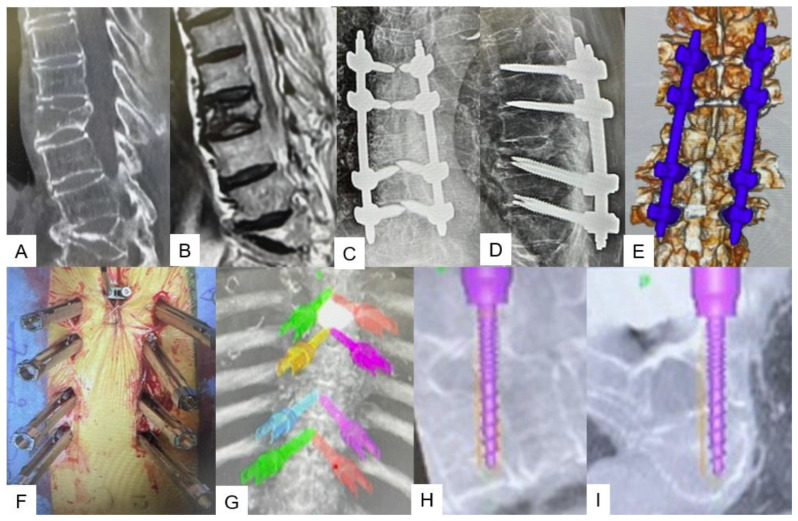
T9 extension fracture. (A): Preoperative midsagittal reconstruction CT, (B): Preoperative midsagittal T2 weighted MRI, (C): Postoperative anteroposterior radiogram, (D): Postoperative lateral radiogram, (E): 3D CT, (F): intraoperative image, (G): 3D image of navigation, (H): Sagittal image of transdiscal screw, (I): Axial image of transdiscal screw.
